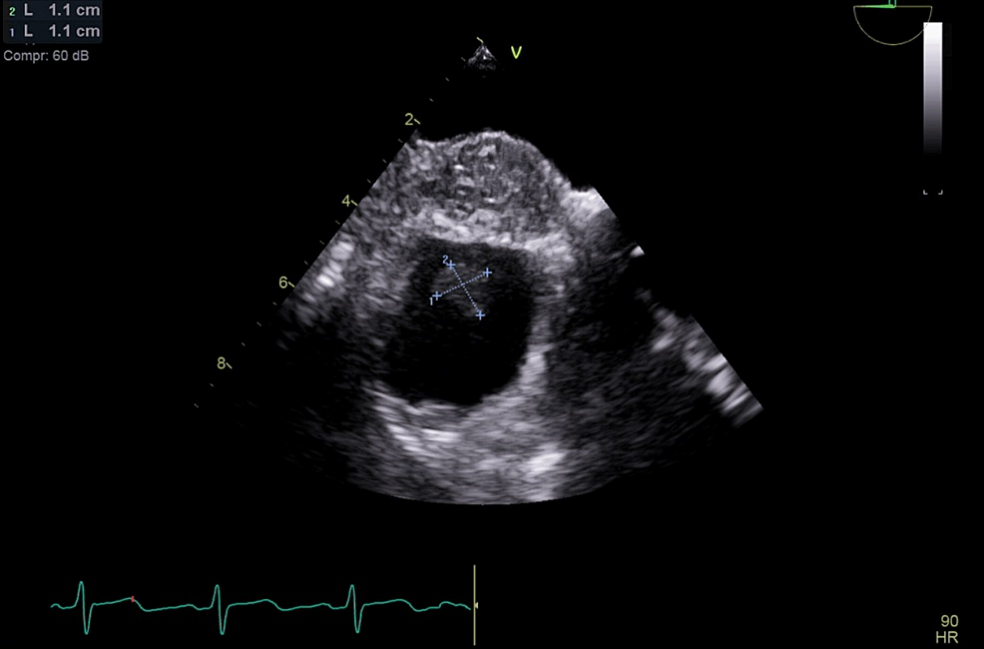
Repeat transesophageal echocardiography after eight weeks revealed a decreasing size of the vegetation
